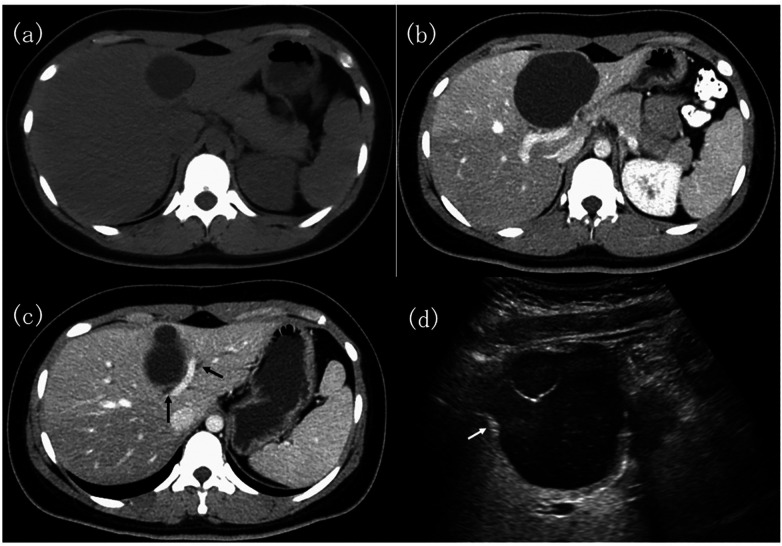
Abdominal computed tomography and ultrasonography findings. (a) Non-contrast CT showing a cystic lesion in the medial segment of the liver. (b) Contrast-enhanced CT demonstrates a multilocular cystic tumor with calcification of the cyst wall. (c) CT image showing dilation of the left hepatic duct and anterior segment bile duct (black arrow). (d) Abdominal ultrasonography demonstrates a multilocular cystic lesion with a small hyperechoic mural nodule (white arrow). Reproduced from Moriuchi et al., Cureus (41), licensed under the Creative Commons Attribution–NonCommercial 4.0 (CC BY-NC 4.0) license, Cureus.
